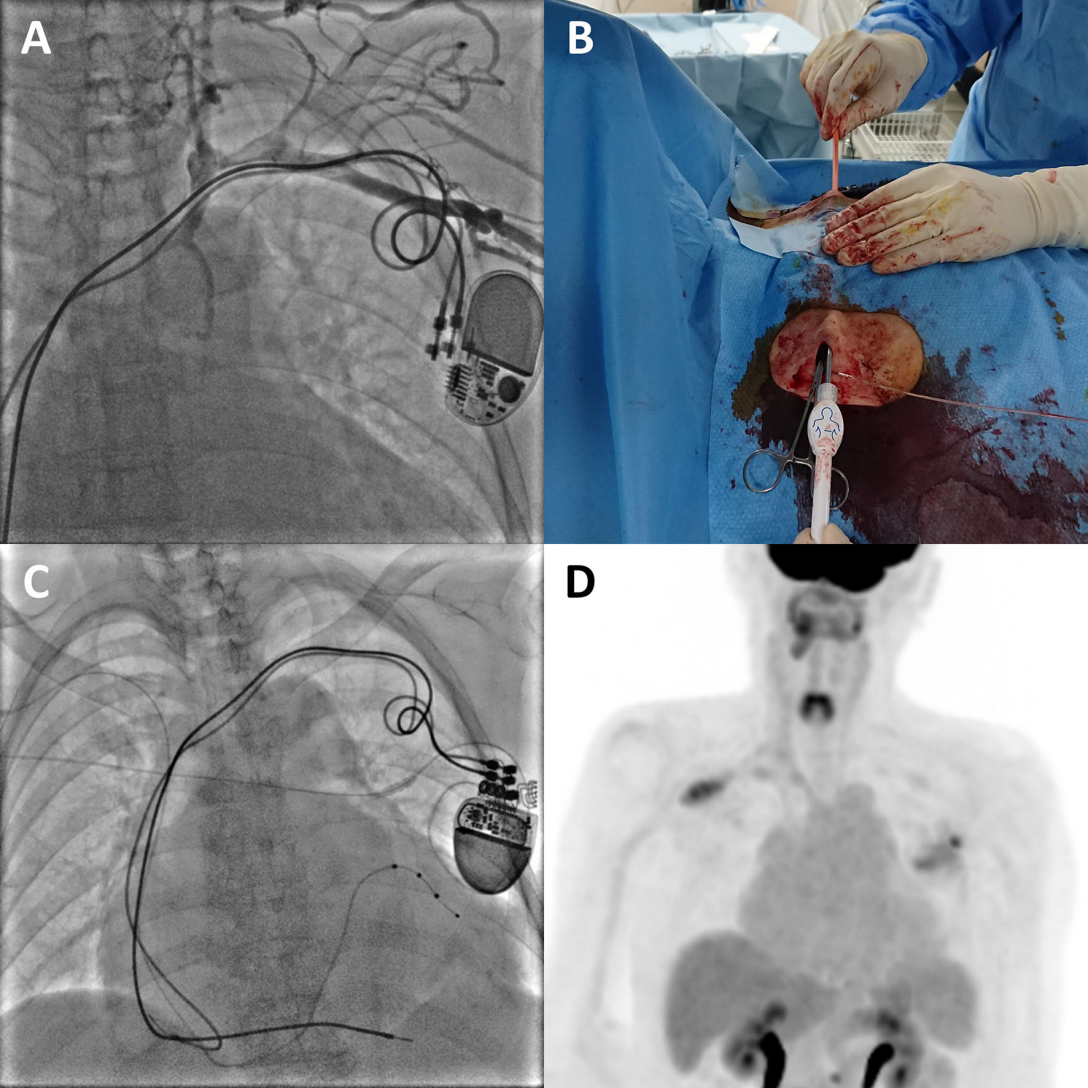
A Venography demonstrates total occlusion of the left brachiocephalic vein with collateral flow drained into the azygos system. B Subcutaneous tunneling of the left ventricular lead from the right to the left pocket of the previous pacemaker. C Chest X-ray after cardiac resynchronization therapy. D
